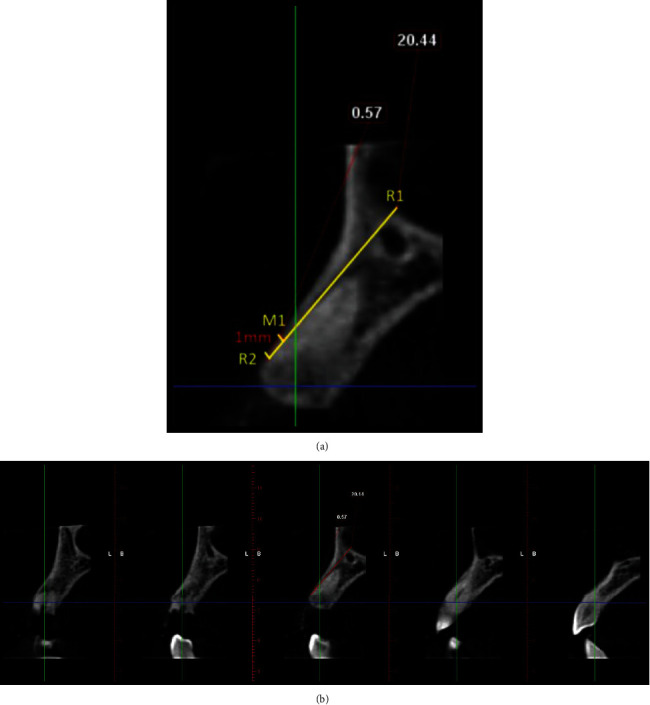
Demonstration of a treated case: radiographic measurements. (a) Preoperative CBCT: sagittal view. (b) Labial cortical thickness assessed using sections of 1 mm thickness.
